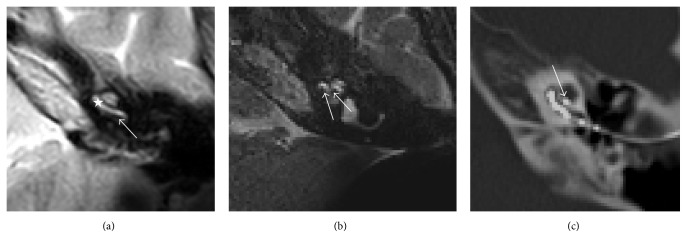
(a) MRI scalar pattern of Patient 8's scala tympani position in the basal turn. The arrow indicates array's diminishing signal in the basal turn. The star indicates the scala vestibuli. (b) MRI scalar pattern of Patient 8's scala tympani position. The arrows indicate the electrodes' positions in the basal and second turns. (c) FPT pattern of Patient 8's scala tympani position. The arrow indicates the scala tympani's position on the floor of the second turn.
